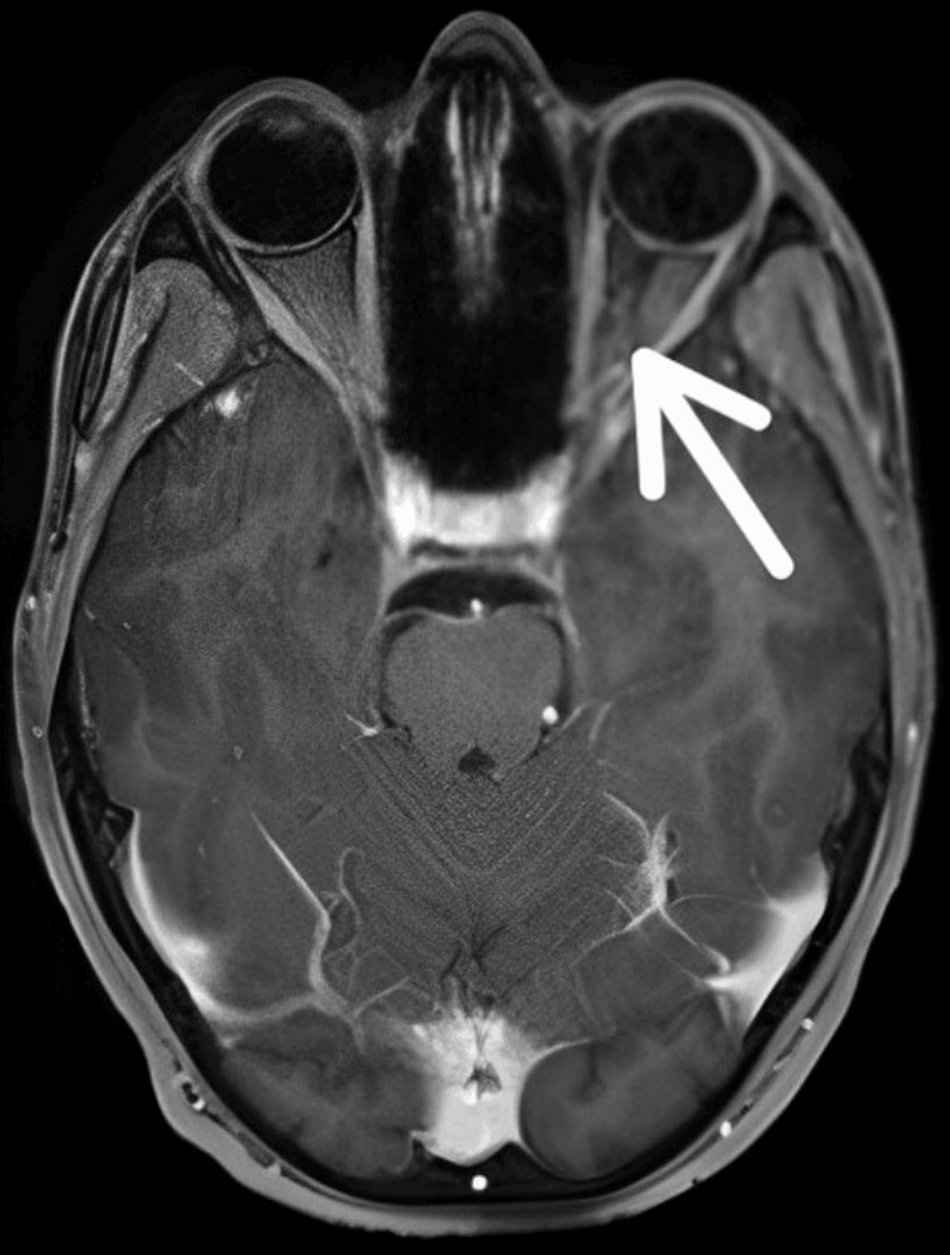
MRI of the brain Arrow indicates hyperintensity on STIR with mild contrast enhancement in the posterior aspects of bilateral optic nerves (intracranial part) with extension till the optic chiasm in optic neuritis MRI: magnetic resonance imaging; STIR: short-tau inversion recovery
